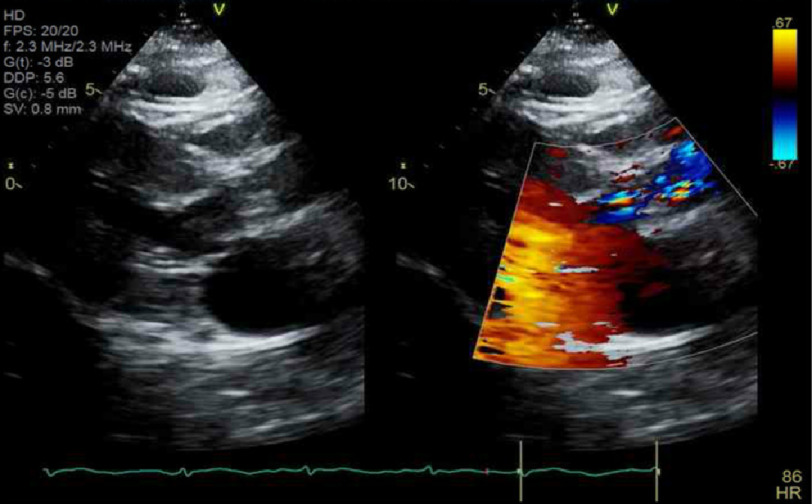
Transthoracic echocardiogram (TTE) showing a heavily calcified aortic valve with severe aortic valve stenosis and an aortic valve area of 0.25 cm^2^.
